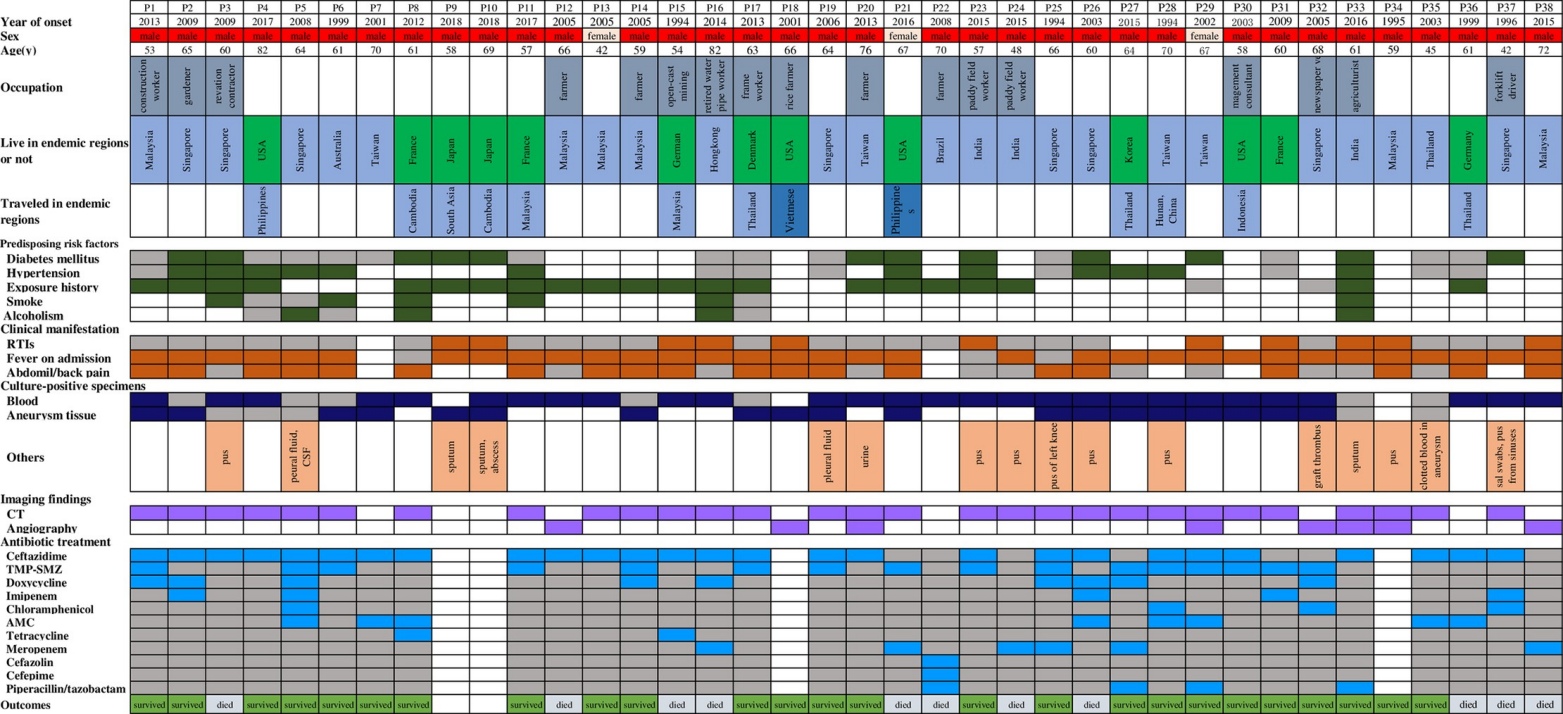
Summary of the 38reported cases of mycotic aneurysm secondary to melioidosis in the literature. RTI: Respiratory tract infections; CT: computed tomography; TMP-SMZ: trimethoprim/sulfamethoxazole; AMC: amoxicillin/clavulanate; Blank cell: data not available; Grey cell: no; other colored cells: yes. Cases, including Case 1 [21], Case 2–3 [4], Case 4 [1],Case 5 [20],Case 6 [22],Case 7 [48],Case 8 [9], Case 9–10 [23], Case 11 [24], Case 12–14 [25], Case 15 [26], Case 16 [3], Case 17 [27], Case 18 [28], Case 19 [29], Case 20 [30], Case 21 [31], Case 22 [19], Case 23–24 [6], and Case 25–26 [10], Case 27 [52], Case 28 [18], Case 29 [32], Case 30 [33], Case 31 [41], Case 32 [35], Case 33 [36], Case 34 [8] Case 35 [37], Case36 [38], Case37 [39], and Case38 [40].
